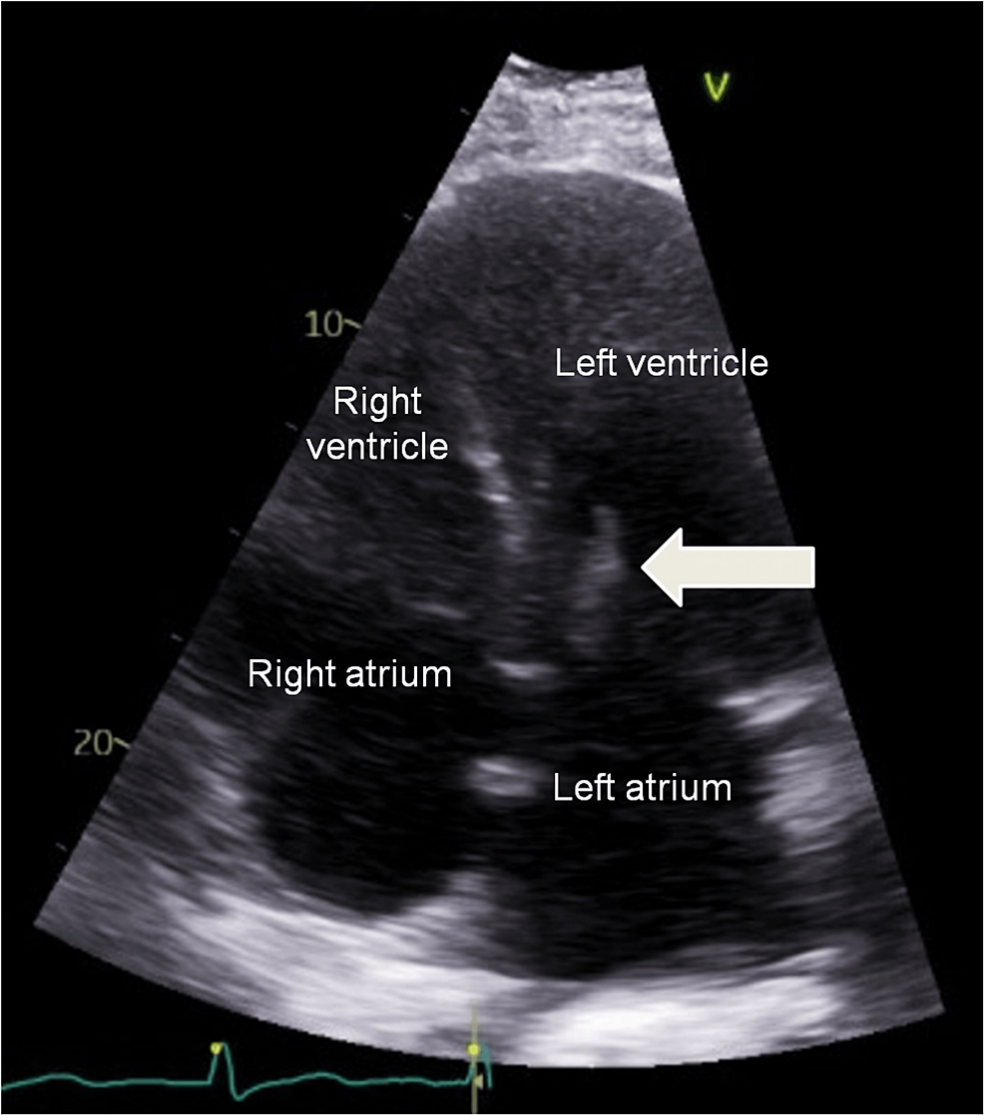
Transthoracic echocardiogram showing mitral valve vegetation, as indicated by the arrow, concerning for endocarditis
